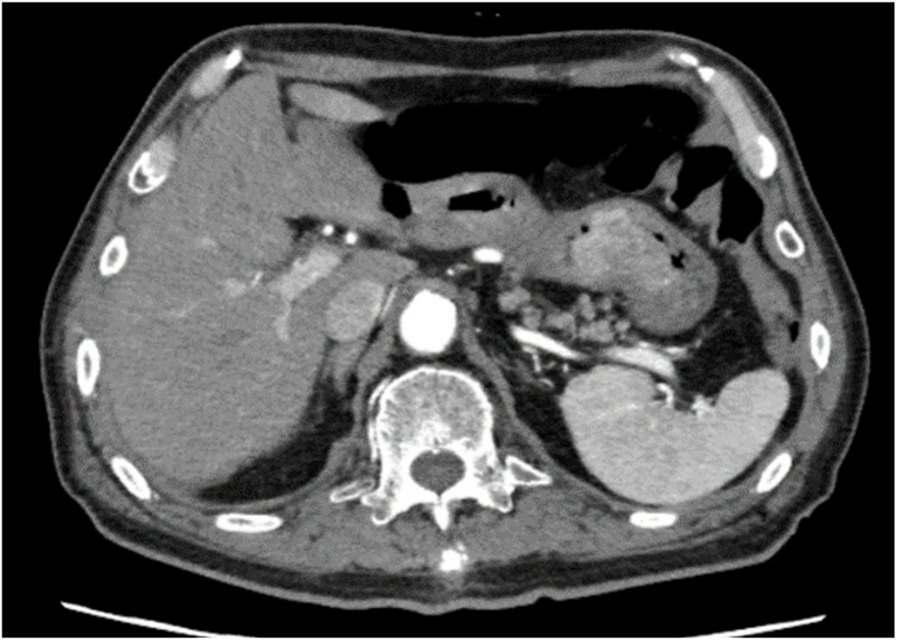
Abdominal computed tomography. Enhanced gastric tumor incarcerated to the antrum
